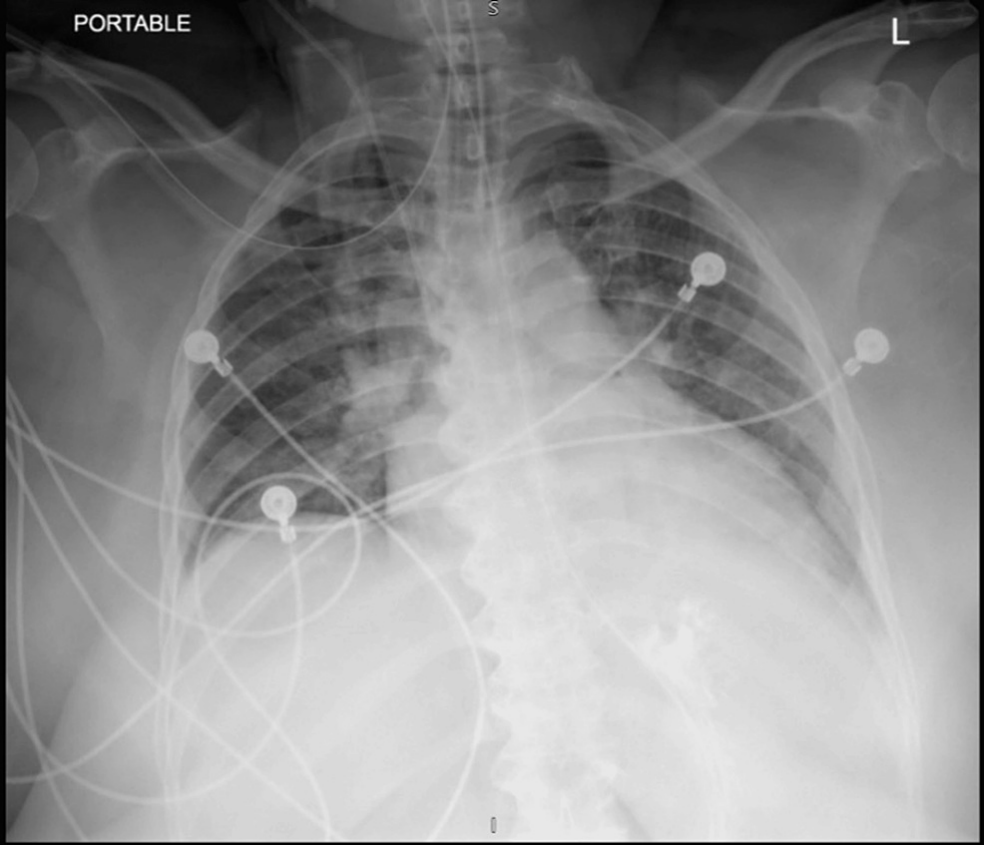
After 48 hours, a chest X-ray with the resolution of diffuse alveolar infiltrates
